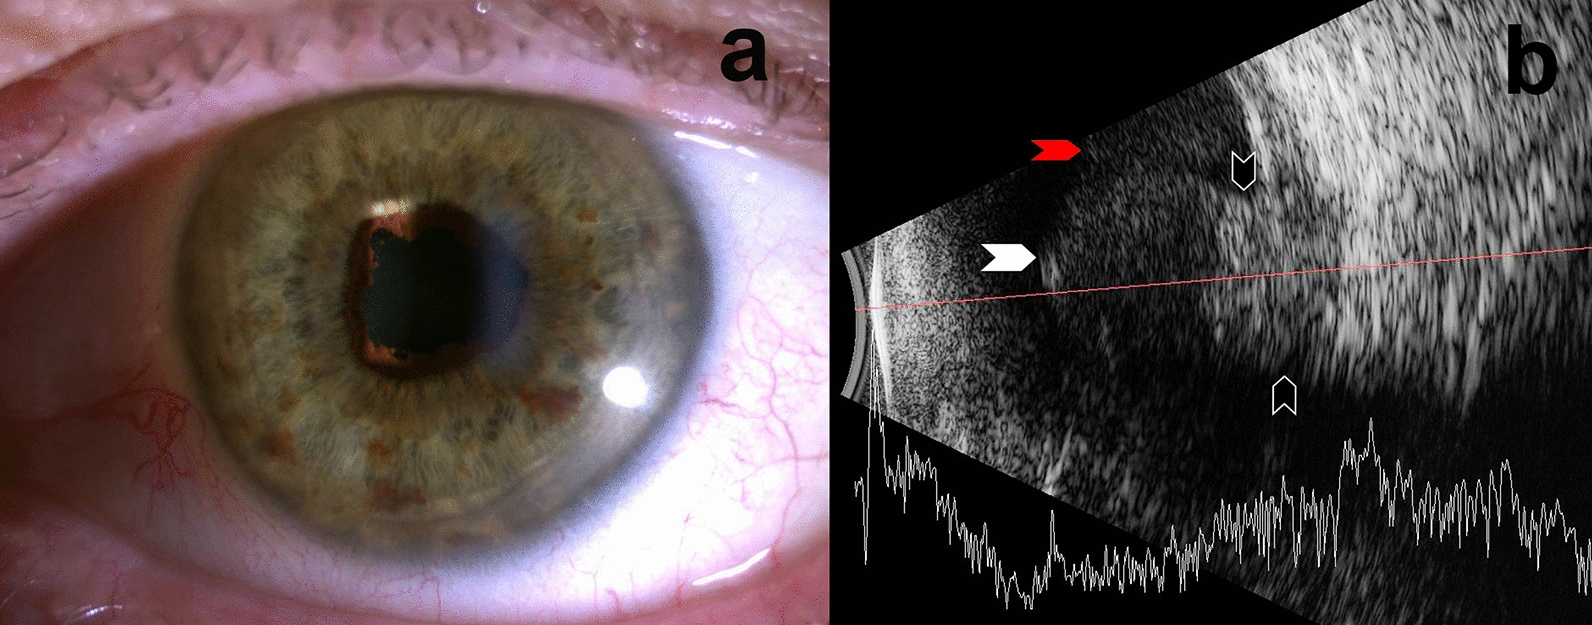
a Anterior segment with posterior synechiae and cataract. b Bscan ultrasound showing posterior segment choroidal mass. Red Arrow: Vitreous hemorrhage, White Arrow: Anterior edge of tumor, Black Arrows: Collar-stud base
